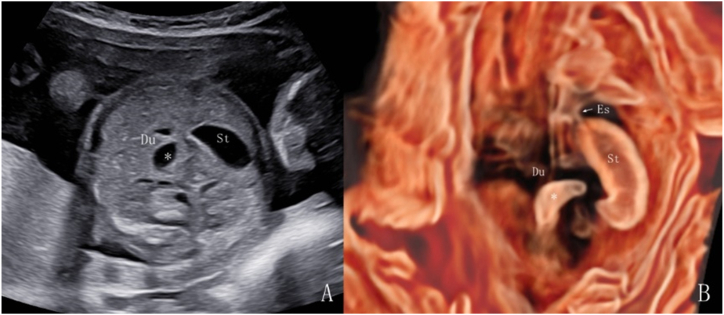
Fetus at 22 weeks and 2 days of gestation with proximal duodenal dilation. Postnatal surgery confirmed proximal narrowing of the descending part of the duodenum. A. 2D ultrasound shows the “double-bubble sign” in the upper abdomen. B. In 3D inversion mode, a clear connection is seen between the esophagus and the gastric bubble, and the proximal duodenal dilation appears as a “crescent moon” shape.
